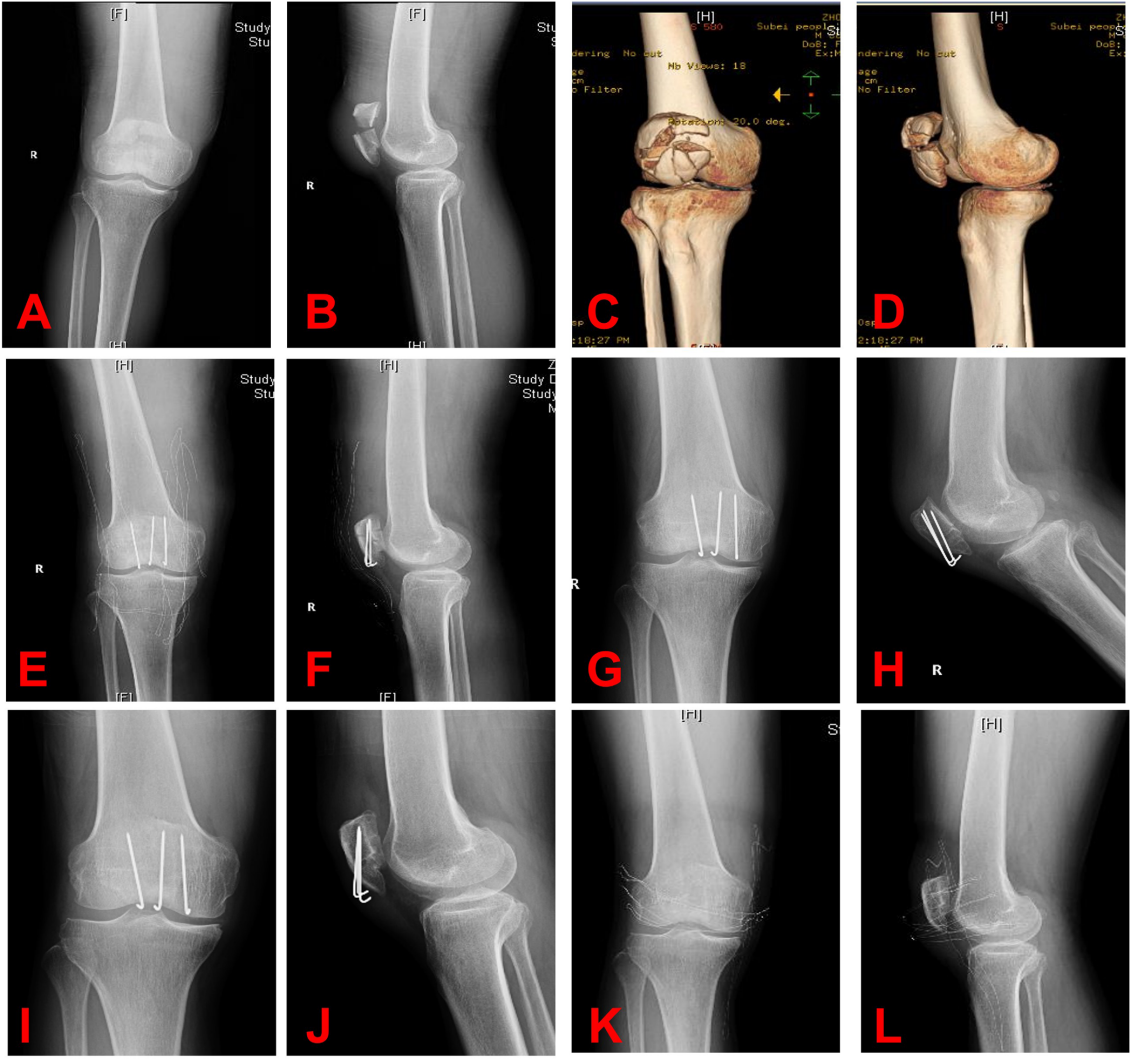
(A–D) Radiographs of comminuted patella fracture. (E,F) In this comminuted patella fracture, we used the 5-Ethibond and three K-wires. (G,H) After 3 months, the radiograph revealed a good union of fracture. (I,J) After 12 months, no breakage, loosening, or migration of the internal fixation was detected, and a good union of fracture was seen. (K,L) Radiograph after implant removal.
